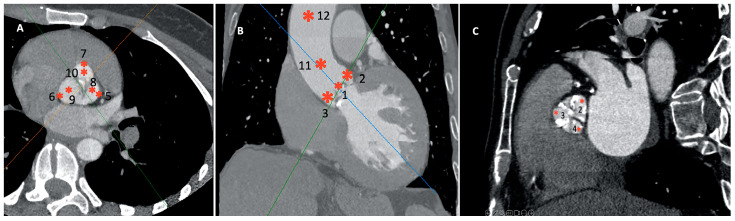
Measurement regions in the CCTA. Regions are as follows: 1: 4–5 mm above the opening of the aortic valve; 2–4: junction of the leaflets and the fibrotic anulus (left, right, and non-coronary); 5–10: mid-level of the sinus of Valsalva at the most lateral and center point of left, right, and non-coronary; 11: sinotubular junction (STJ); and 12: 4 cm from the SJT. (A,C) are oblique, (B) is the frontal view. From (C), crosshair is removed for better view. (CCTA: cardiac computed tomography angiography).
